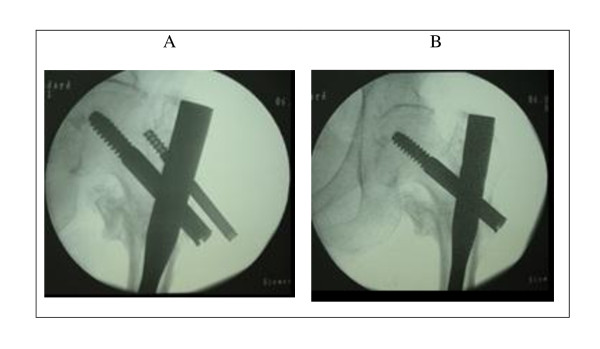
Case 3 - Radiographies of the left hip joint showing the correct consolidation of the left intertrochanteric femoral fracture (A), together with the lateral migration of the superior screw while the sliding screw remained in the original position. The removal of the superior screw is shown in (B).
